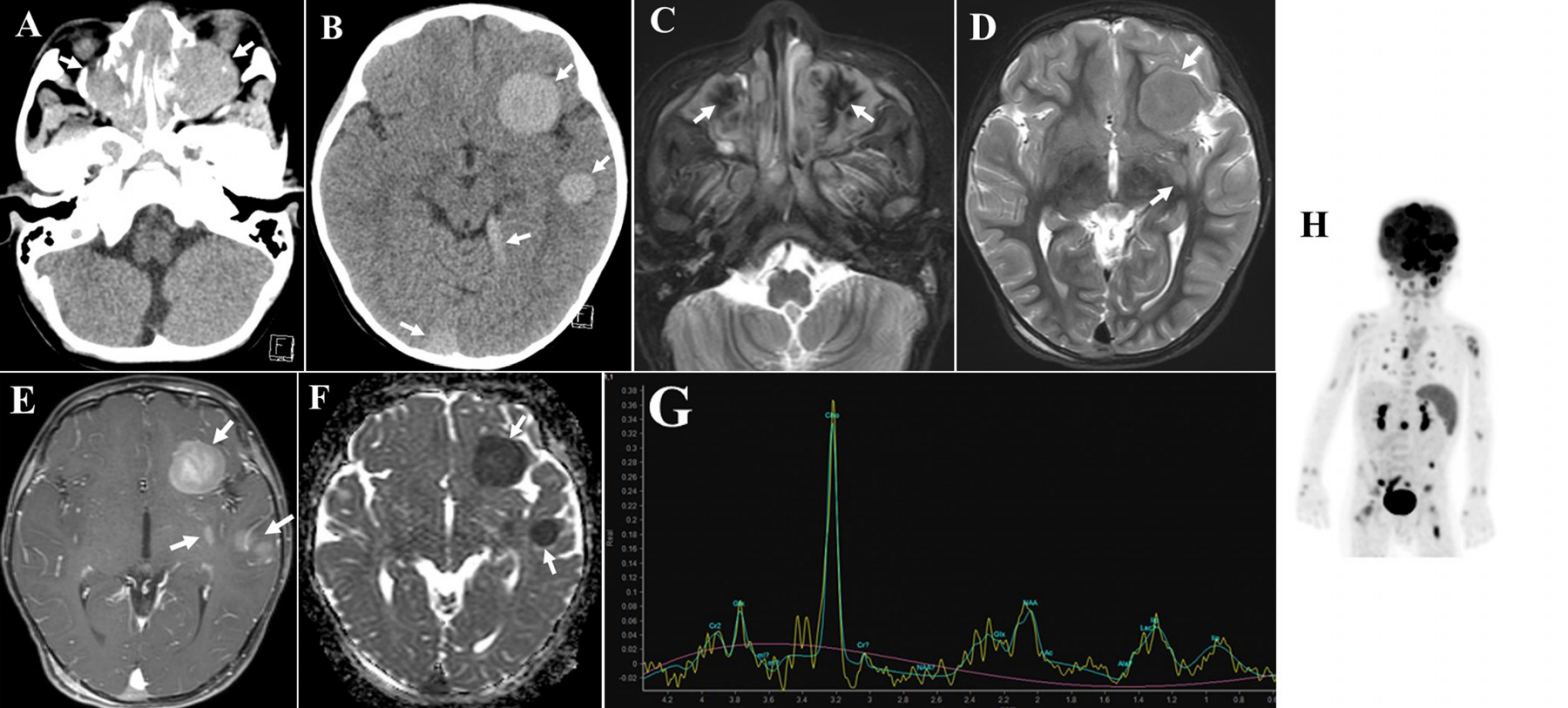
Plain CT images show bilateral nasal and paranasal soft tissue masses with bony destruction (A: arrows) and multiple discrete hyperdense masses in either intra-axial or extra-axial brain (B: arrows). STIR images show the low signal intensity in the middle of maxillary sinus lesions (C: arrows) and isointense masses with no edema in the brain (D: arrows). Contrast-enhanced T1-weighted image shows multiple homogeneously enhancing masses (E: arrows). ADC map shows restricted diffusion of the masses (F: arrows). MRS shows increased choline peak and the presence of lipid and lactate (G). FDG-PET shows multiple uptakes of the lesions (H).
